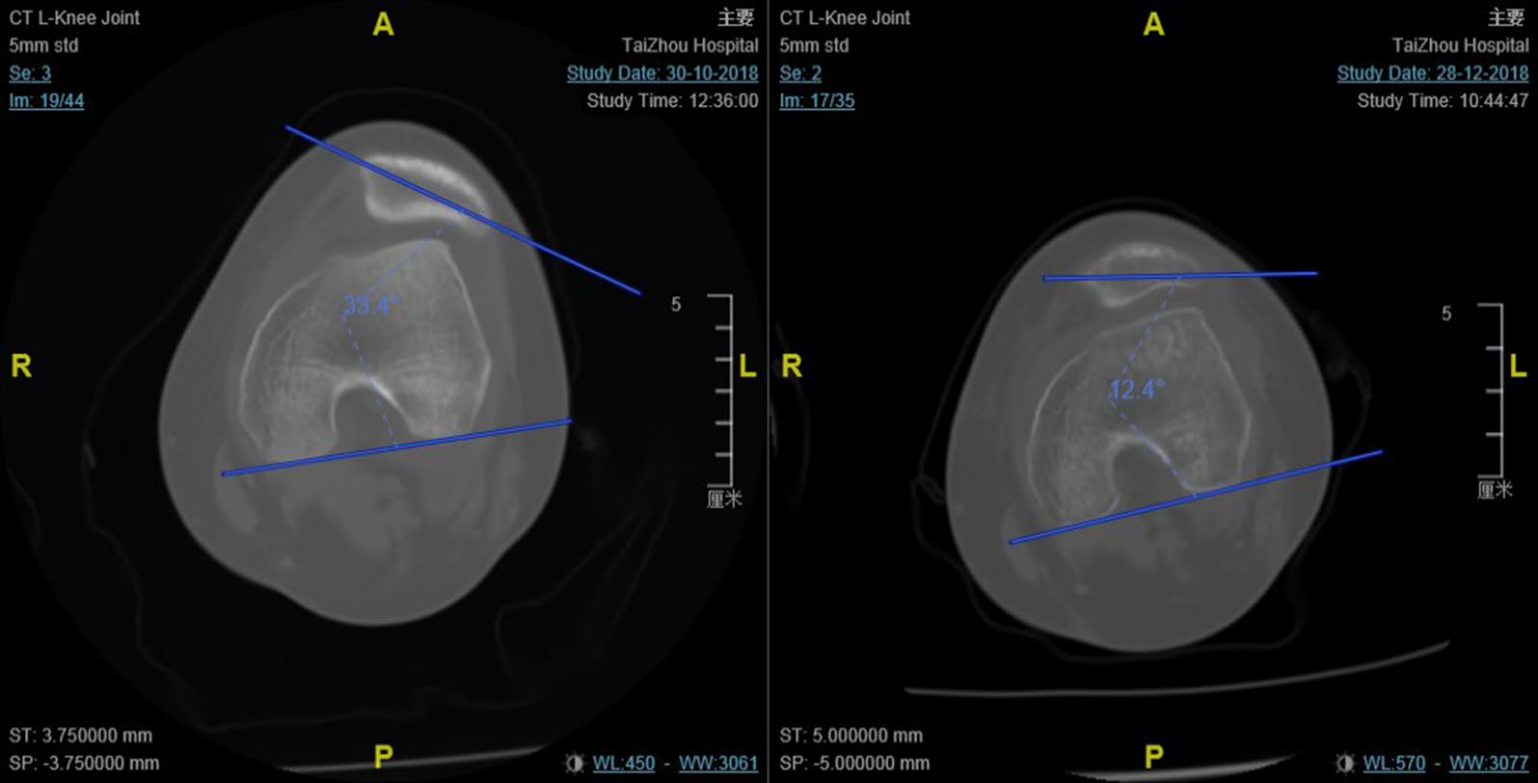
Preoperative and postoperative computerized tomography (CT) scans of the patellar tilt angle (the angle between the femoral posterior condylar line and the line defining the maximal patellar width)
